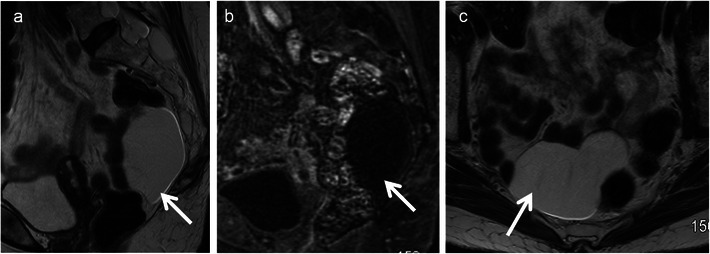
Retrorectal or tailgut cyst in a 42-year-old woman. a Sagittal T2-weighted MRI reveals unilocular cystic lesion (*arrow*) in the retrorectal location. b Sagittal T1-weighted MRI image reveals the lesion (*arrow*) to be hypointense. c Axial T2-weighted MRI again demonstrates the cystic lesion (*arrow*)
